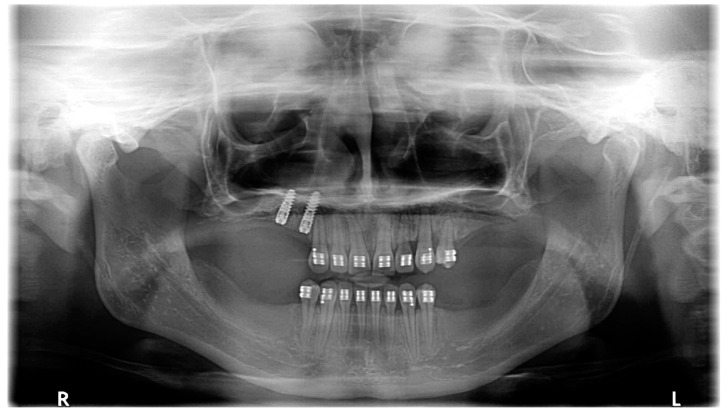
Panoramic radiograph performed during the finishing phase.
